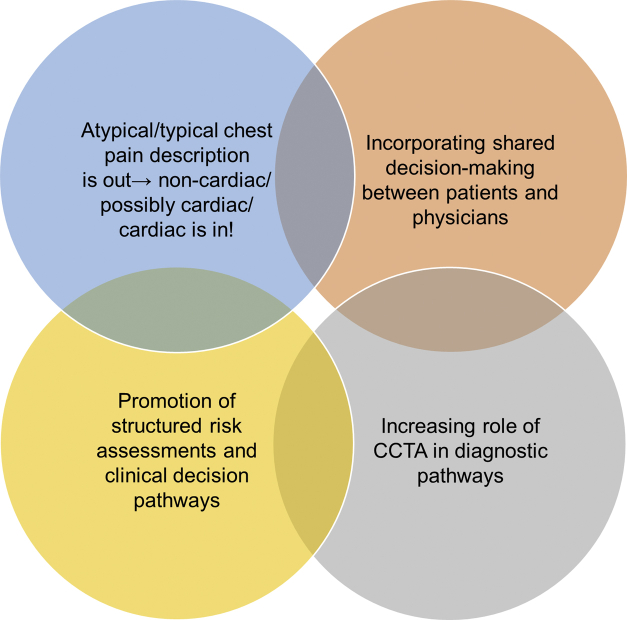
Central themes for the 2021 American Heart Association/American College of Cardiology guideline for the evaluation and diagnosis of chest pain. CCTA, coronary computed tomography angiography.
